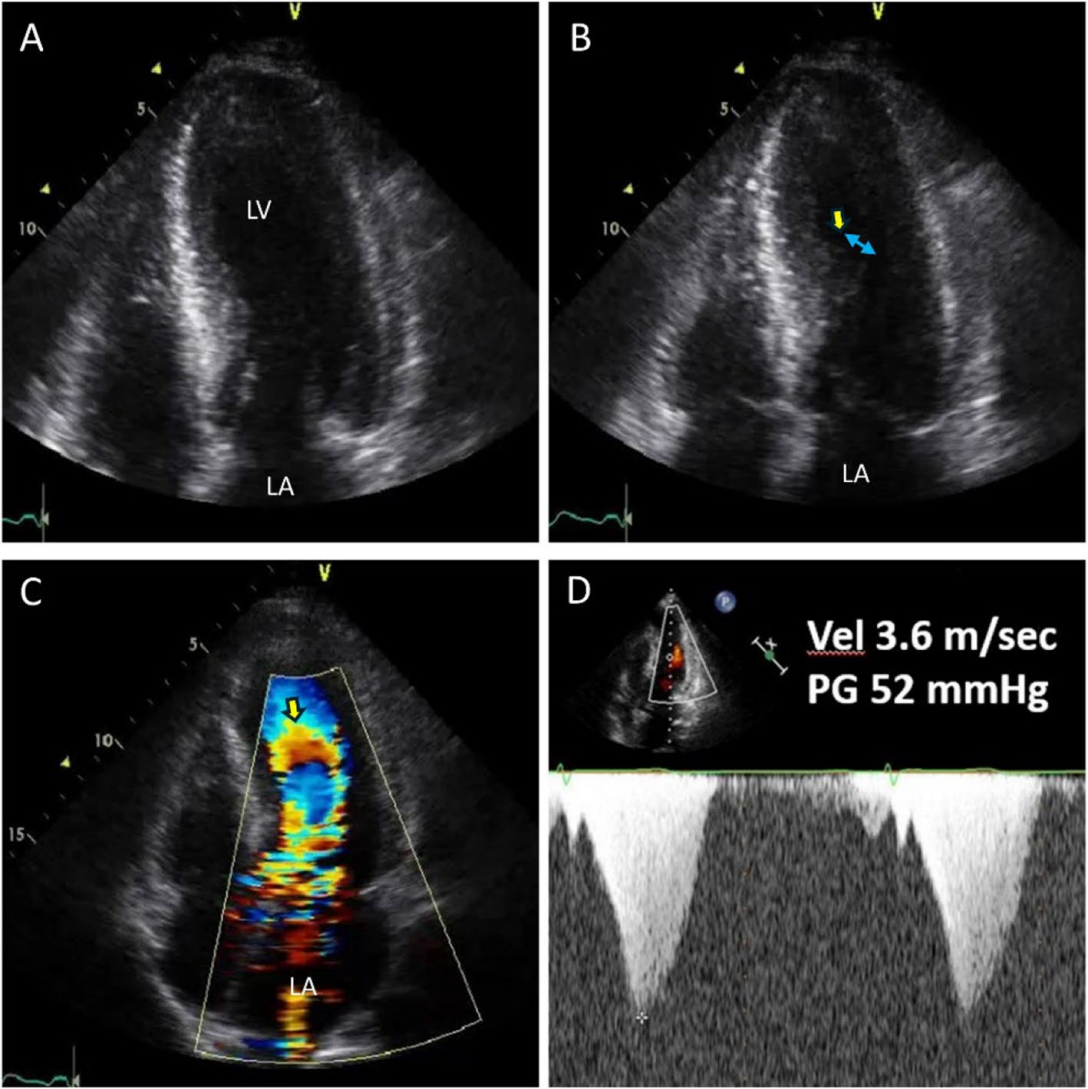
Representative echocardiographic images of midventricular obstruction. During systole, (A, B) the hypertrophied septum and thick papillary muscle come into contact, which (C) induces flow acceleration from the left ventricular midcavity. D Doppler tracing shows a typical dagger-shaped increased systolic flow velocity with a delayed peak
